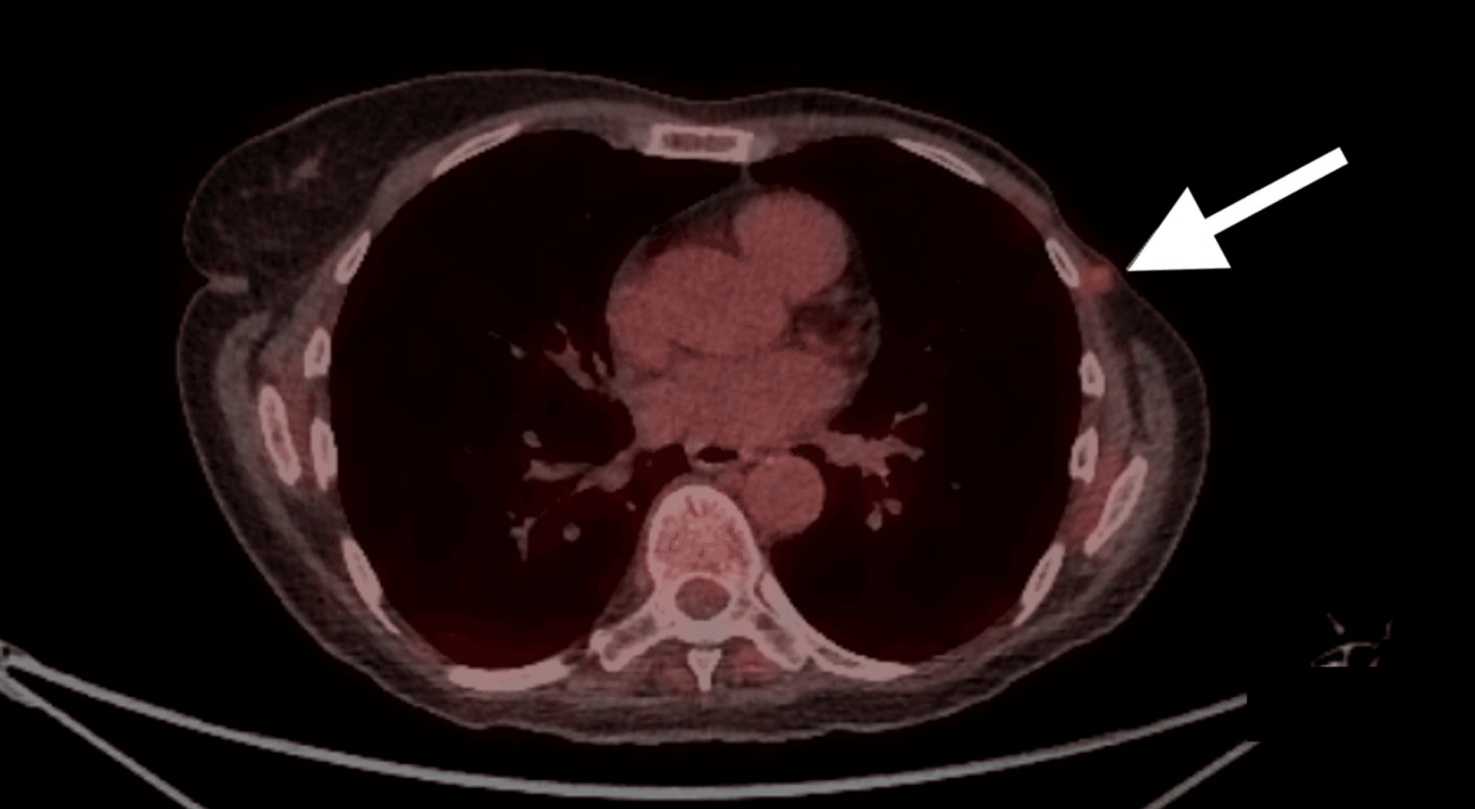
Arrow pointing at subcutaneous soft tissue nodule (0.7 cm) at left mastectomy bed, exhibiting low FDG uptake in PET scan (white arrow). PET: positron emission tomography, FDG: fluorodeoxyglucose.
